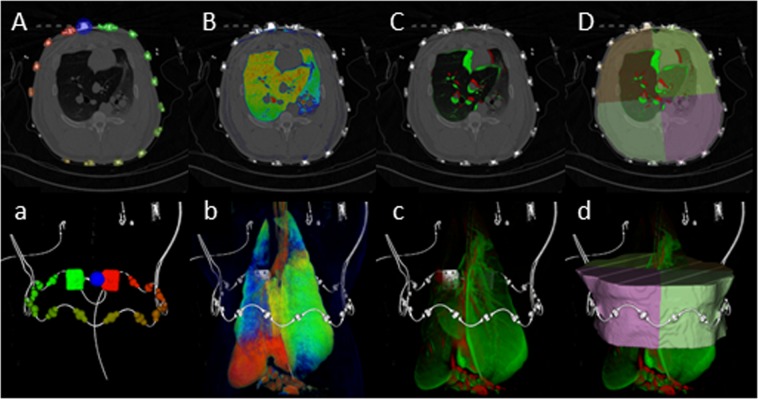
Automatic process of coregistration shown by axial slices view (capital letters A–D) and volume renderings (a–d): Step 1 consists of identification of the EIT belt electrodes in the CT datasets (A,a), which can be identified in the EIT data as well. Then pulmonary density maps (B,b) of inspiration and expiration were calculated. Consecutively, difference maps (C,c) were computed. Finally, analysis was virtually restricted to the volume next to the belt (D,d), which was called ΔDECT_(Belt)_.
